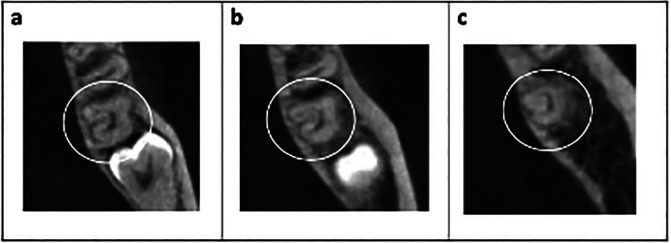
CBCT images of a C-molar with the same C1 configuration: (a) coronal third, (b) middle third, and (c) apical third.
